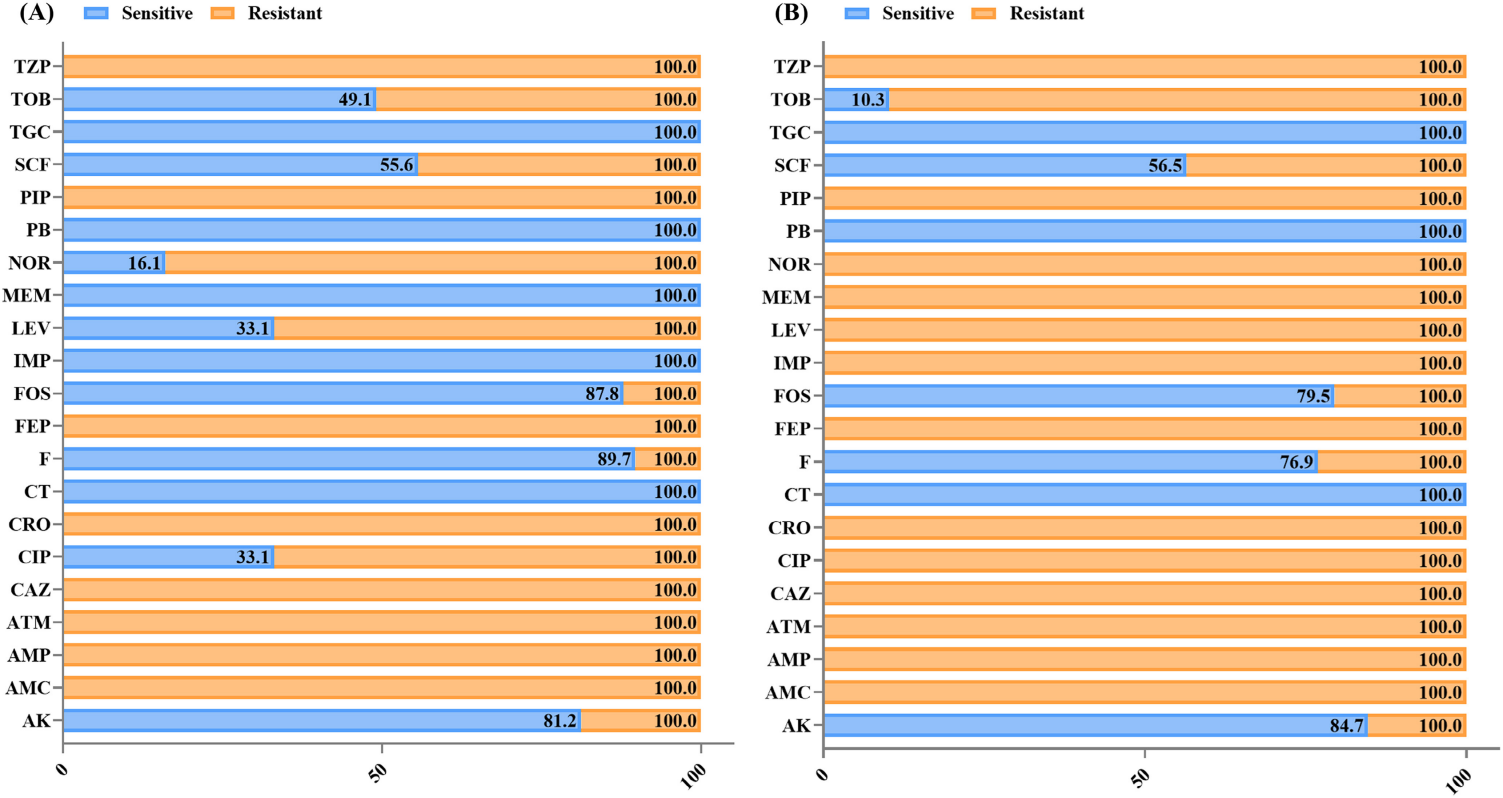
Antibiogram of *Klebsiella pneumoniae* clinical isolates, featuring color‐coded zones to underscore their antibiotic sensitivity or resistance. (A) The AMR characteristics of ESBL producers; (B) the AMR profile of carbapenem‐resistant isolates. AK, amikacin; AMC, amoxicillin/clavulanic acid; AMP, ampicillin; ATM, aztreonam; CAZ, ceftazidime; CIP, ciprofloxacin; CRO, ceftriaxone; CT, colistin; F, nitrofurantoin; FEP, cefepime; FOS, fosfomycin; IMP, imipenem; LEV, levofloxacin; MEM, meropenem; NOR, norfloxacin; PB, polymyxin B; PIP, piperacillin; TGC, tigecycline; TOB, tobramycin; TZP, piperacillin/tazobactam.
